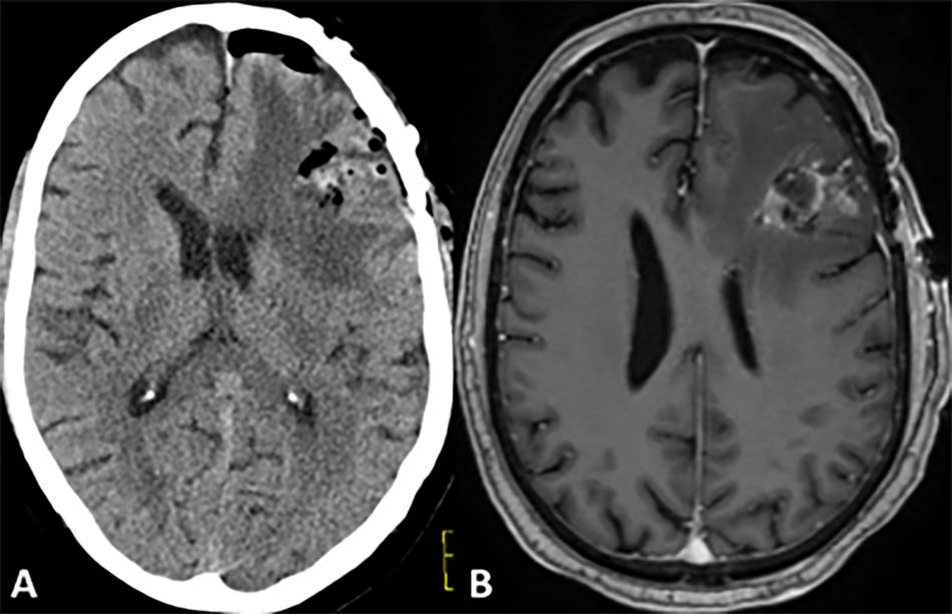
Postoperative CT and MRI scans of the head indicating complete tumor resection with reduced midline shift.
